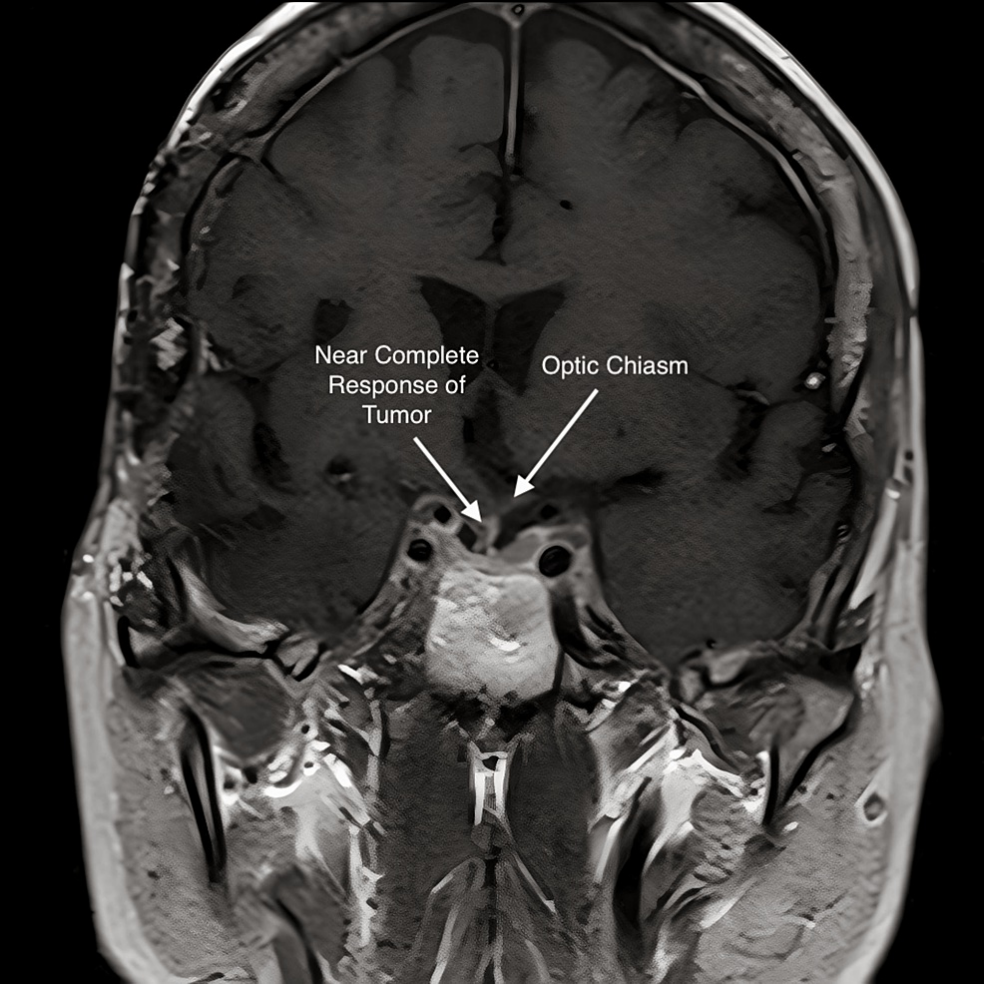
Mid-coronal MRI with contrast; the seven-month post-treatment scan shows a near-complete response to treatment
